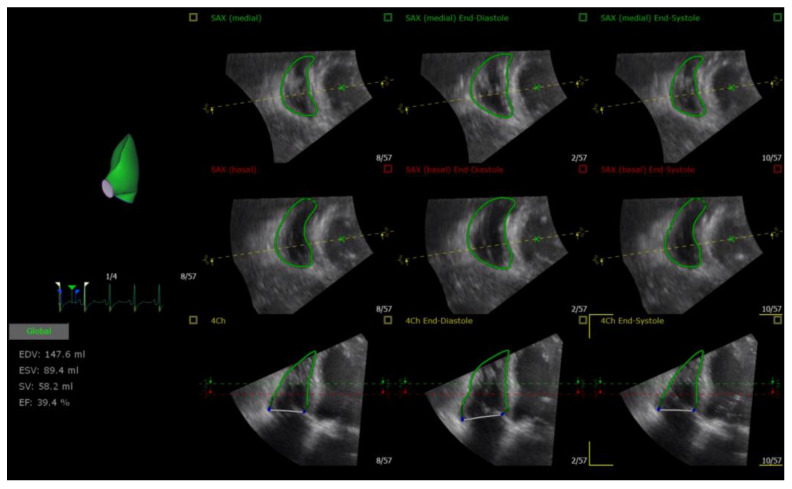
The 3D RV echocardiographic measurements.
